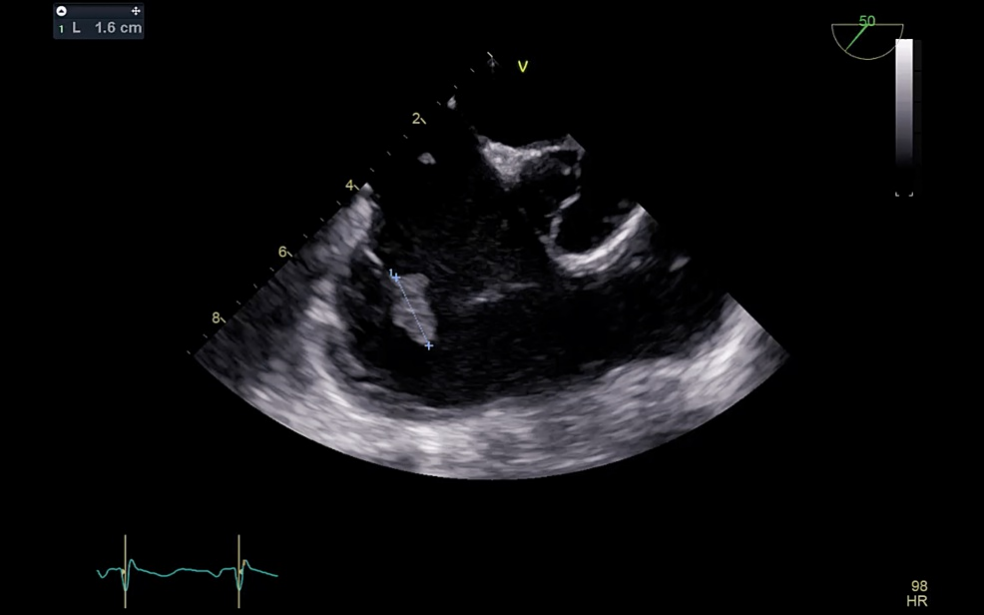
A mid-esophageal short access view of the first transesophageal echocardiogram at the level of the aortic valve showing a large mass (dashed line) attached to the anterior and posterior tricuspid valve leaflets.
